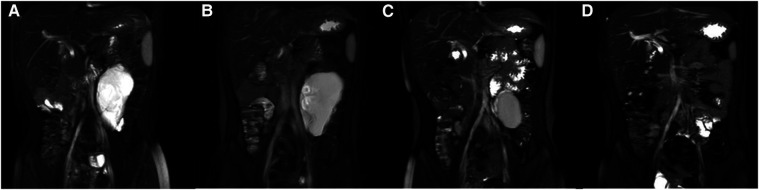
Pre- and posttreatment changes of lesions on MRI (Case 1). (A) Pretreatment MRI. (B) Posttreatment MRI 1 month after the first sclerotherapy. (C) Posttreatment MRI 6 months after the second sclerotherapy. (D) Posttreatment MRI 1 year after diagnosis.
